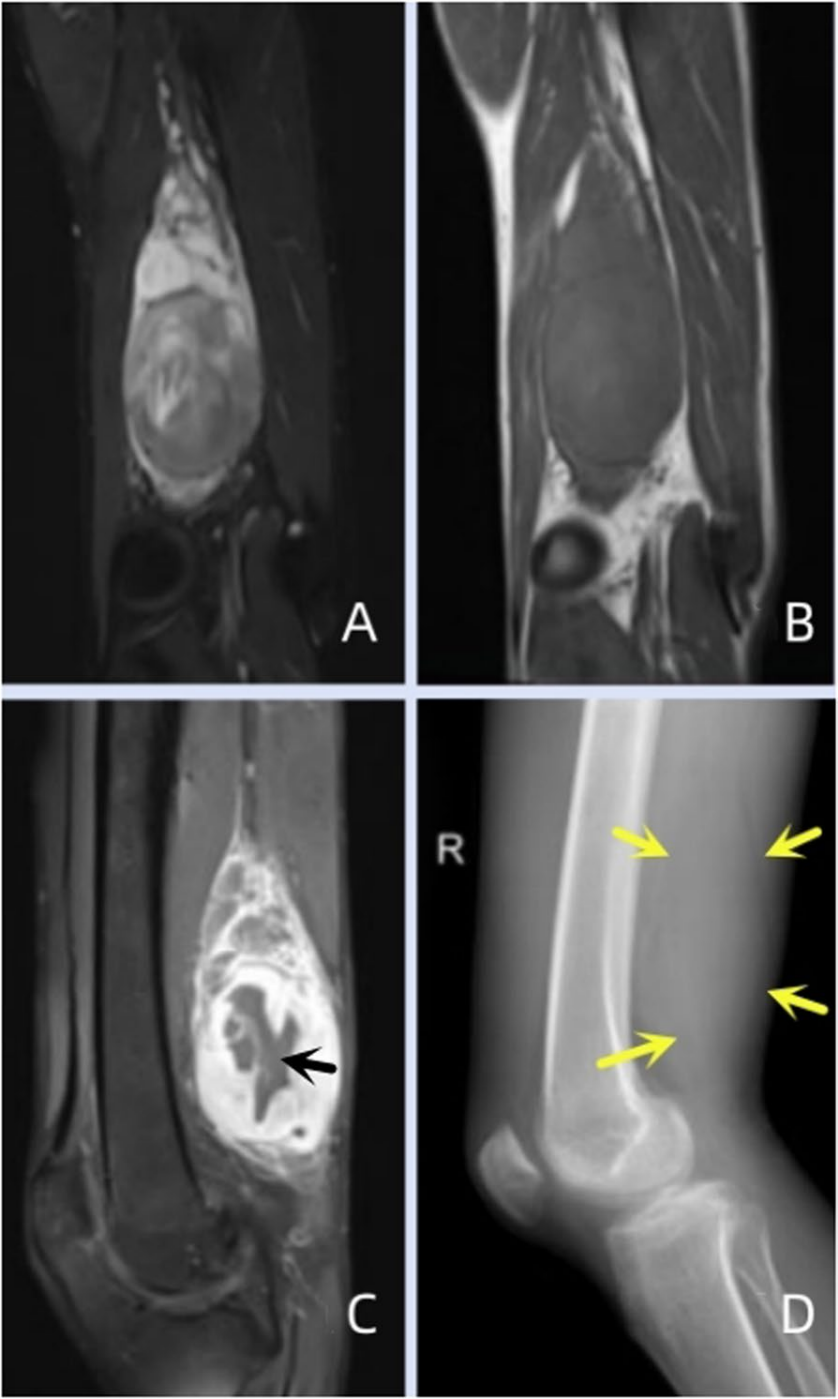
A 23-year-old man with EOS in the right thigh. A, B MR plain scan showing a mass with clear borders behind the femur in the lower thigh. The T1WI signal was similar to the muscle signal, and the T2WI signal was mixed. The parenchymal component had a slightly high signal, and the cystic part had a significantly high signal. C Enhanced MR scan showing progressively marked enhancement of the parenchymal component without enhancement in the cystic area (black arrow). D X-ray showing a mass resembling a muscle density mass (yellow arrow) without obvious calcification or ossification
